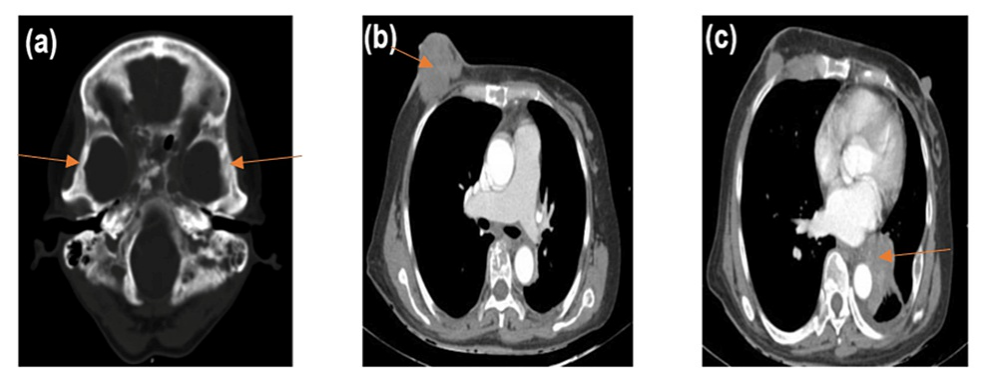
CT images acquired in the axial plane demonstrating (A) temporal bony metastases, (B) a fungating right breast mass, and (C) lung metastases.
